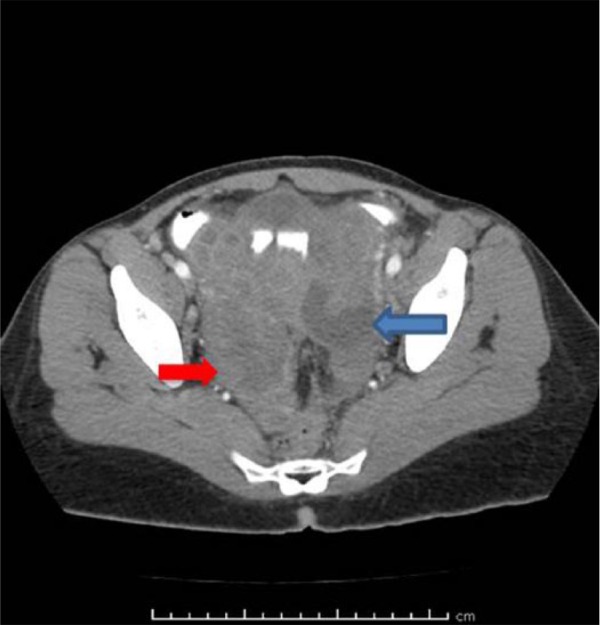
CT of the abdomen with contrast, transverse view: large hypodense bilateral pelvic mass.
